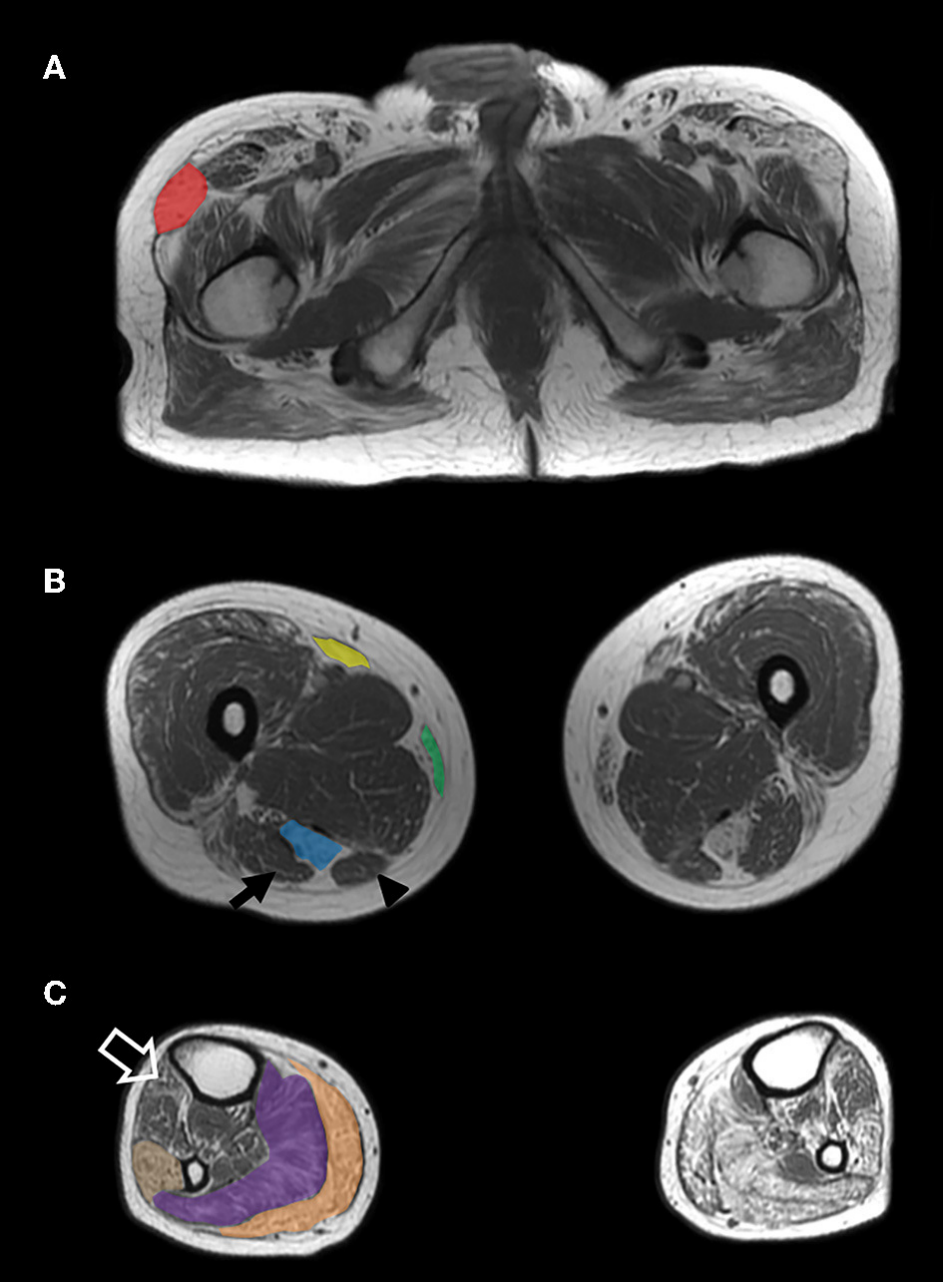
MRI findings. Axiat T1-weighted MRI images of the proximal thigh (A), largest diameter of the mid-thigh (B) and largest diameter of the mid-calf (C) demonstrate grade IV (>50% of the muscle) atrophy and fatty replacement of the tensor fasciae latae (A—red), semitendinosus (B—blue), sartorius (B—yellow), gracilis (B—green), gastrocnemius (C—orange), soleus (C—purple), and peroneus longus (C—tan) muscles. The biceps femoris (B—arrow), semimebranosus (B—arrowhead), and tibialis anterior (C—open arrow) are relatively spared.
